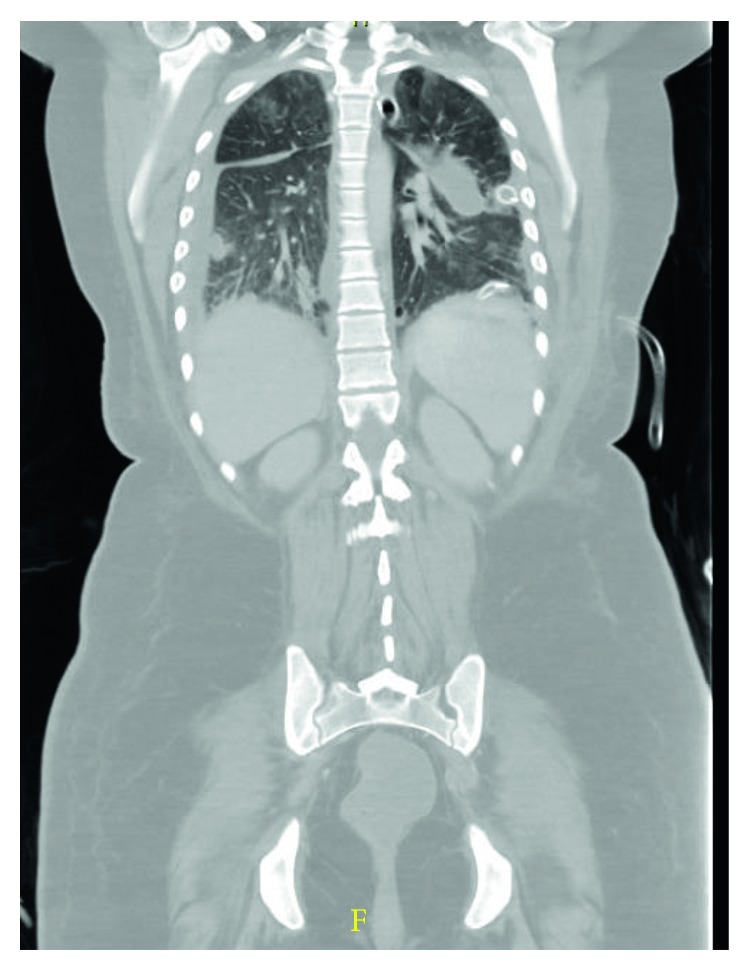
Repeat CT chest abdomen pelvis with contrast 5 days later showing septic emboli in the lungs bilaterally and bilateral loculated empyema.
